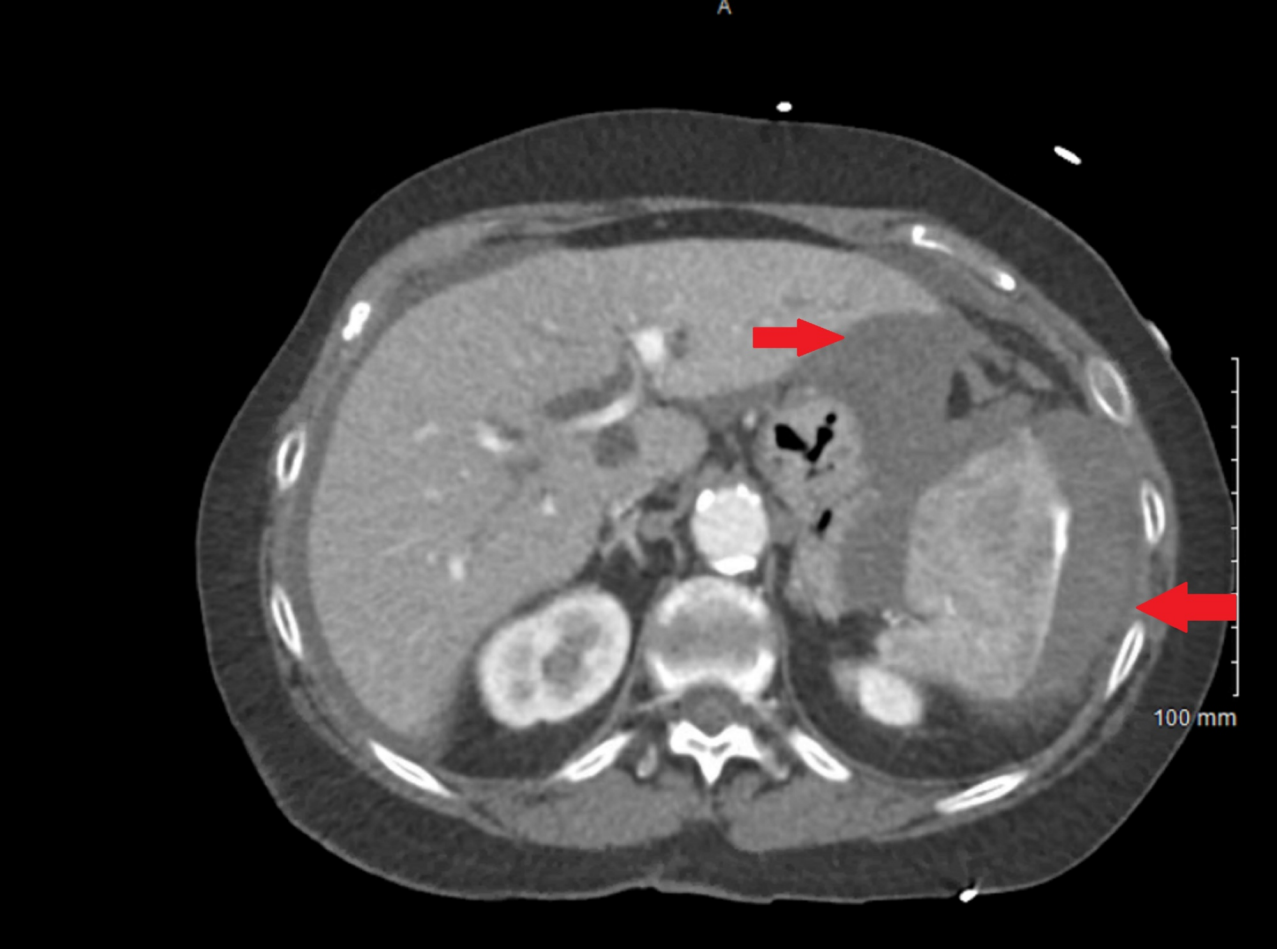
Contrast-enhanced abdominal computed tomography, red arrows showing perisplenic fluid
